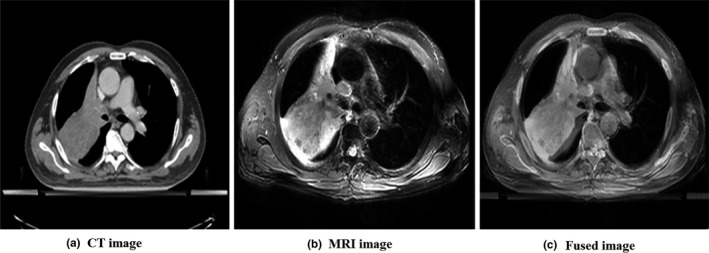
Image registration based on the spine. (a) CT image, (b) MRI image, and (c) fused image.
